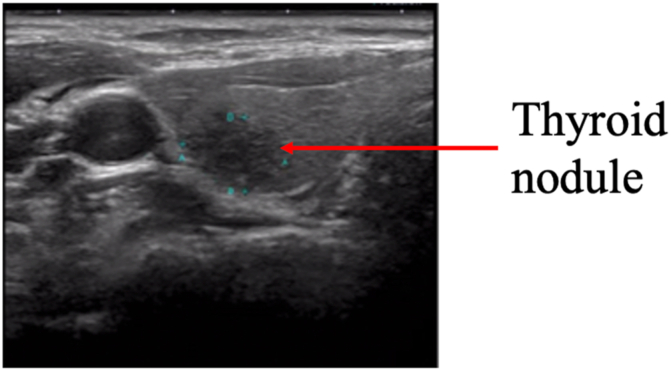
Ultrasound image of the *right* thyroid lobe obtained in 2016, demonstrating a hypoechoic nodule in the *lower* pole (1.3 × 0.9 × 0.6 cm) prior to fine-needle aspiration.
